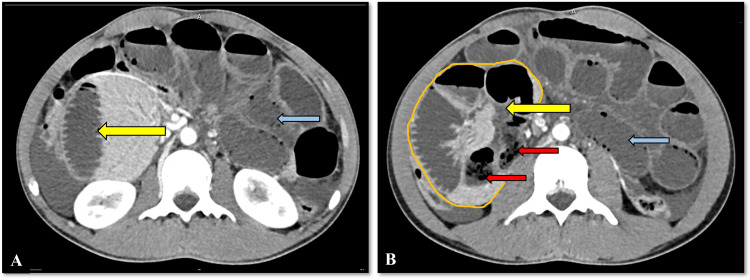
Right paraduodenal hernia Axial sections of the CECT abdomen (A, B) show a cluster of dilated distal duodenal and proximal jejunal loops on the right side of the abdomen (yellow arrows). B: A few small bowel loops in the hernial sac show a small bowel feces sign (red arrows). Also note the dilated small bowel loops in the rest of the abdominal cavity (blue arrows). CECT: contrast-enhanced computed tomography
